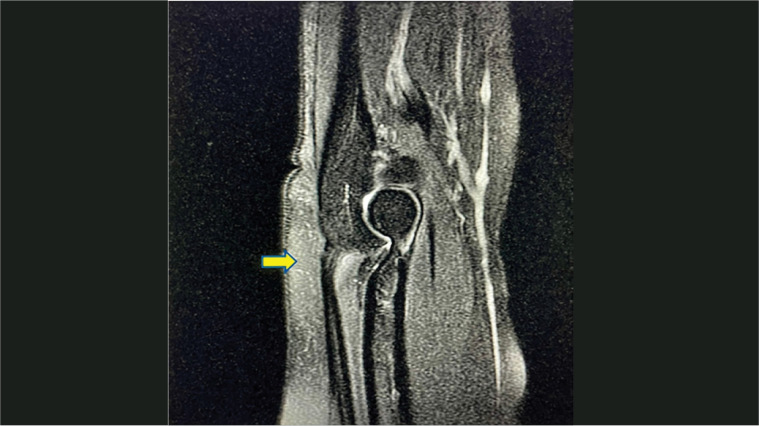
T2 Sequence MRI sagittal scan showing the distal triceps tendon insertion in the olecranon (yellow arrow).
